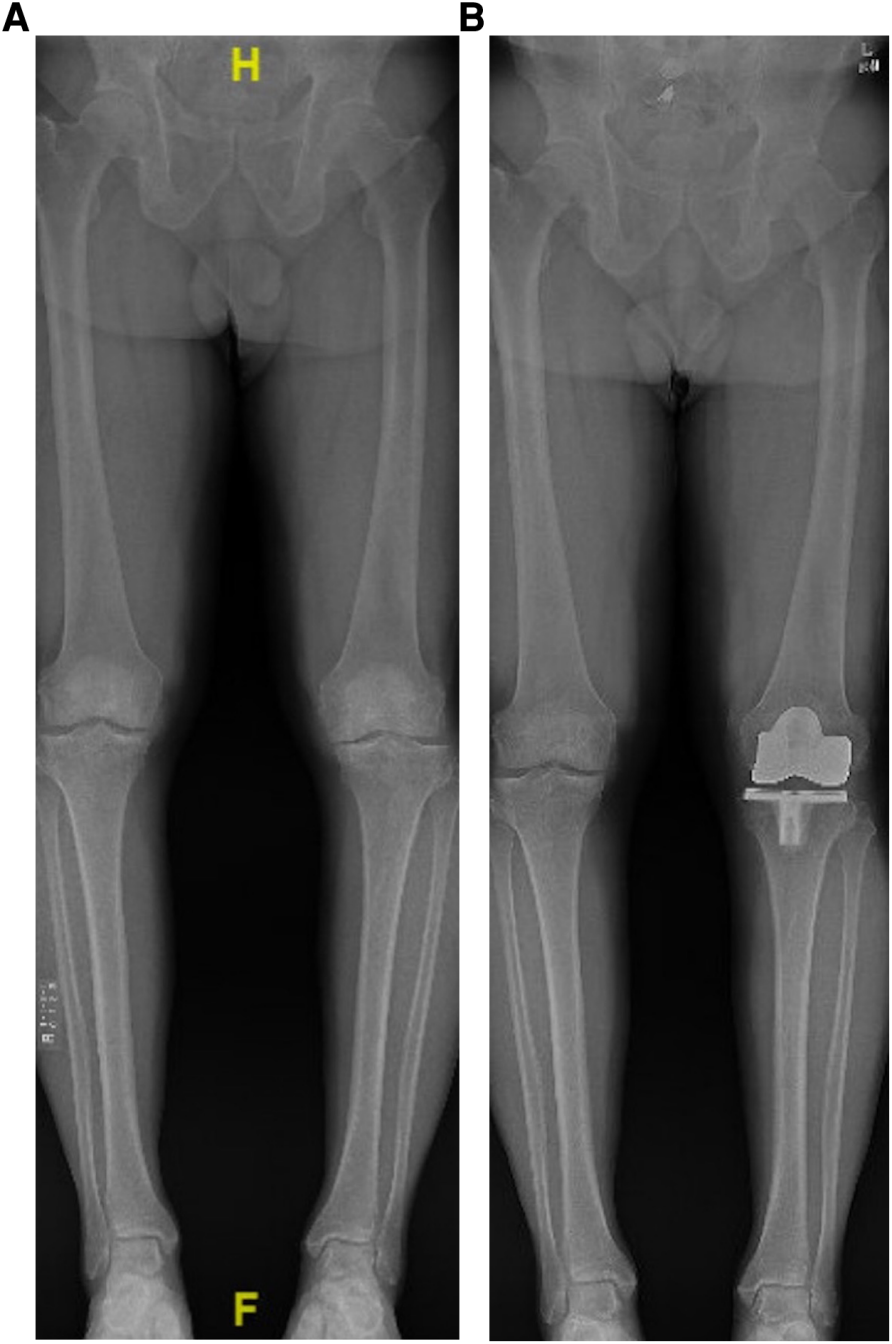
(A) example of pre-operative standing long-leg radiograph showing constitutional varus of the right lower extremity and varus malalignment of the left lower extremity secondary to end-stage osteoarthritis. (B) Post-operative standing long-leg leg radiograph using functional alignment to achieve slight varus alignment without any ligamentous releases.
